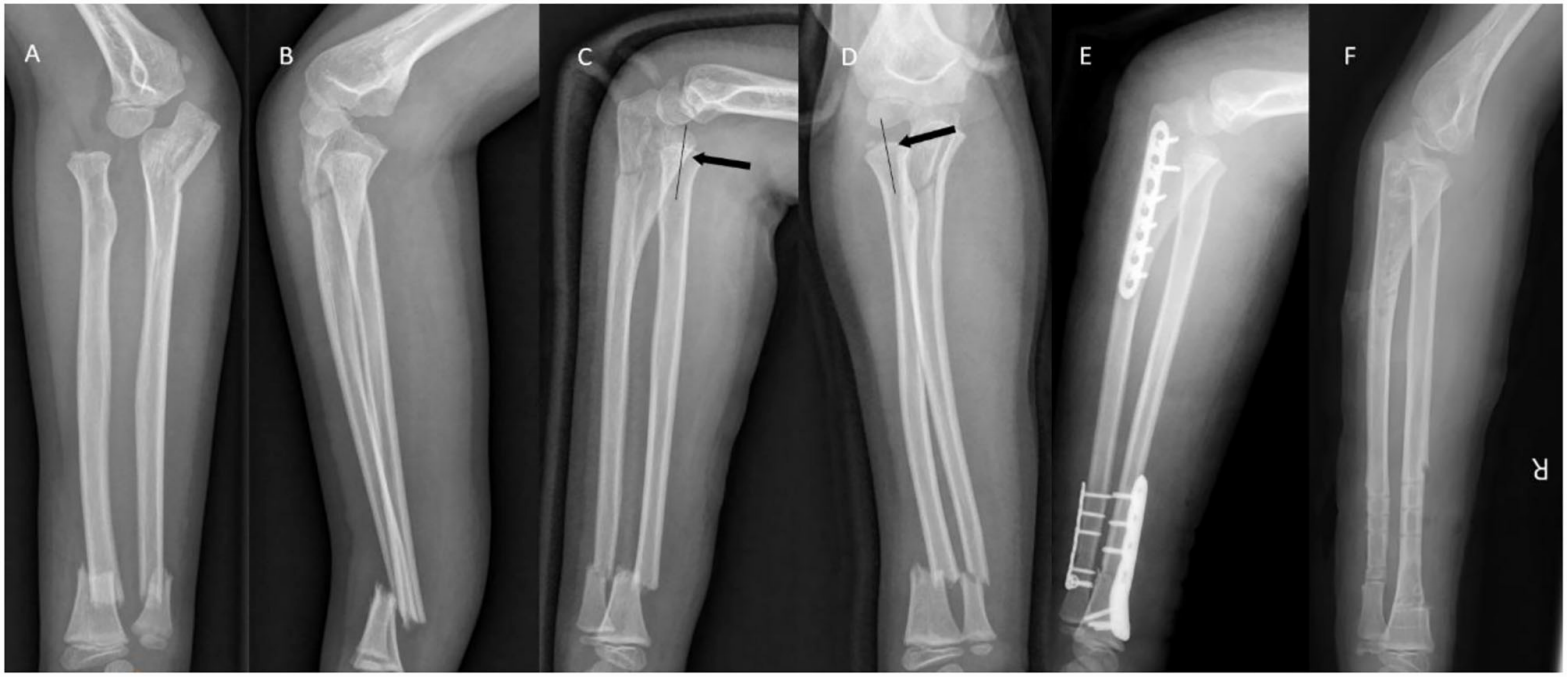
(A,B) X-ray images at initial examination. Bado type III Monteggia fracture and ipsilateral distal forearm fracture were observed. (C,D) Following initial traction, the radial axis did not pass the midpoint of the humeral capitulum (black arrow). (E) Intraoperative radiographs demonstrated good alignment of the fracture. (F) One year after surgery.
